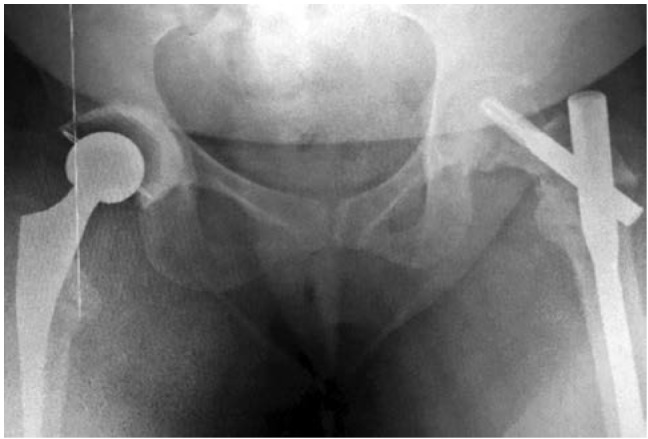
Pelvis AP radiograph showing right total hip arthroplasty and left proximal femoral nail.
